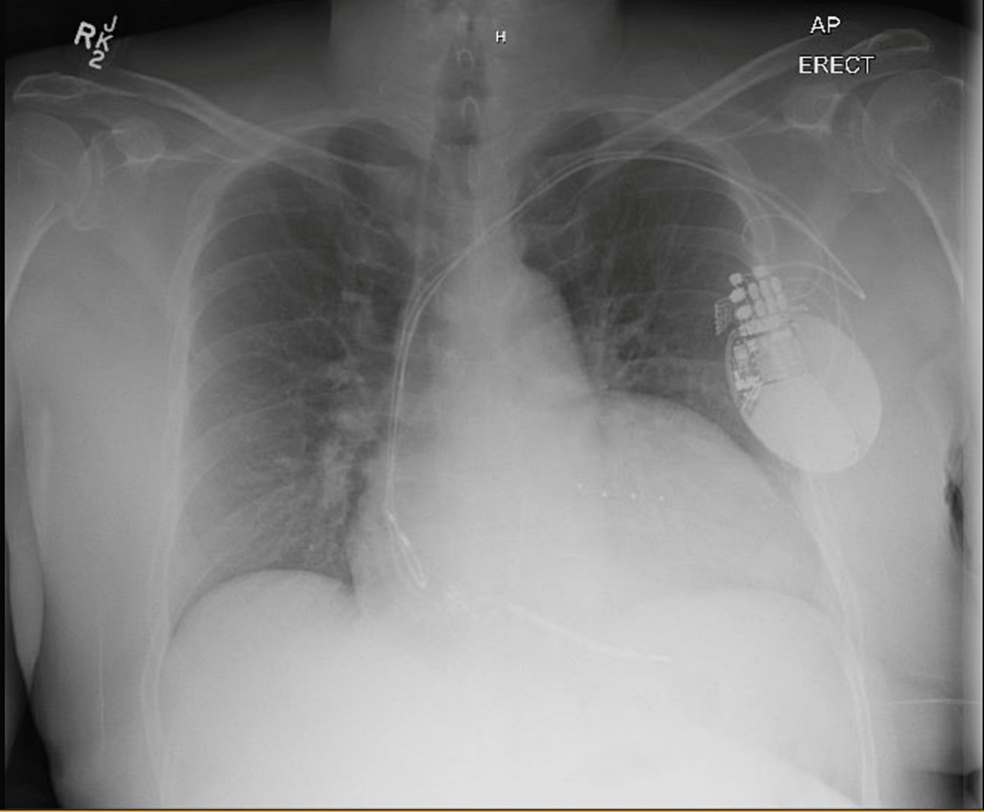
Chest radiograph showing an enlarged heart and an automated intracardiac defibrillator. There is no focal consolidation, pulmonary vascular congestion, pleural effusion, or pneumothorax
